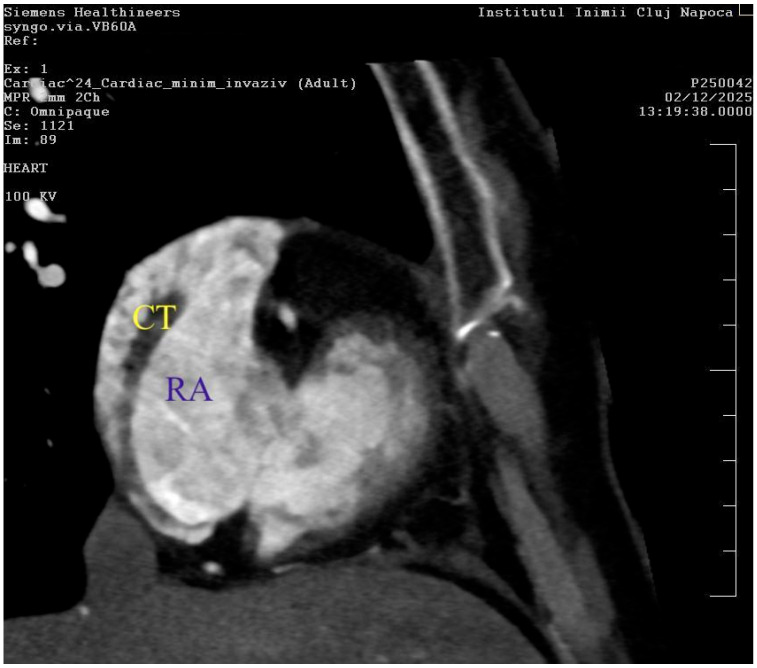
Cardiac computed tomography angiography (CCTA) demonstrates a non-enhancing intracavitary mass within the right atrium, presenting as a well-circumscribed filling defect. CT: crista terminalis, RA: right atrium.
